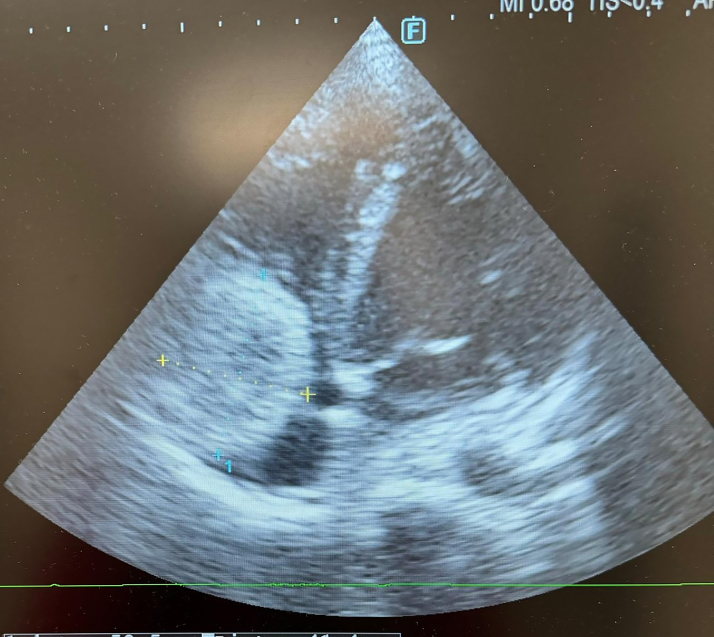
Echocardiographic Image of a Mass in the Right Atrium
